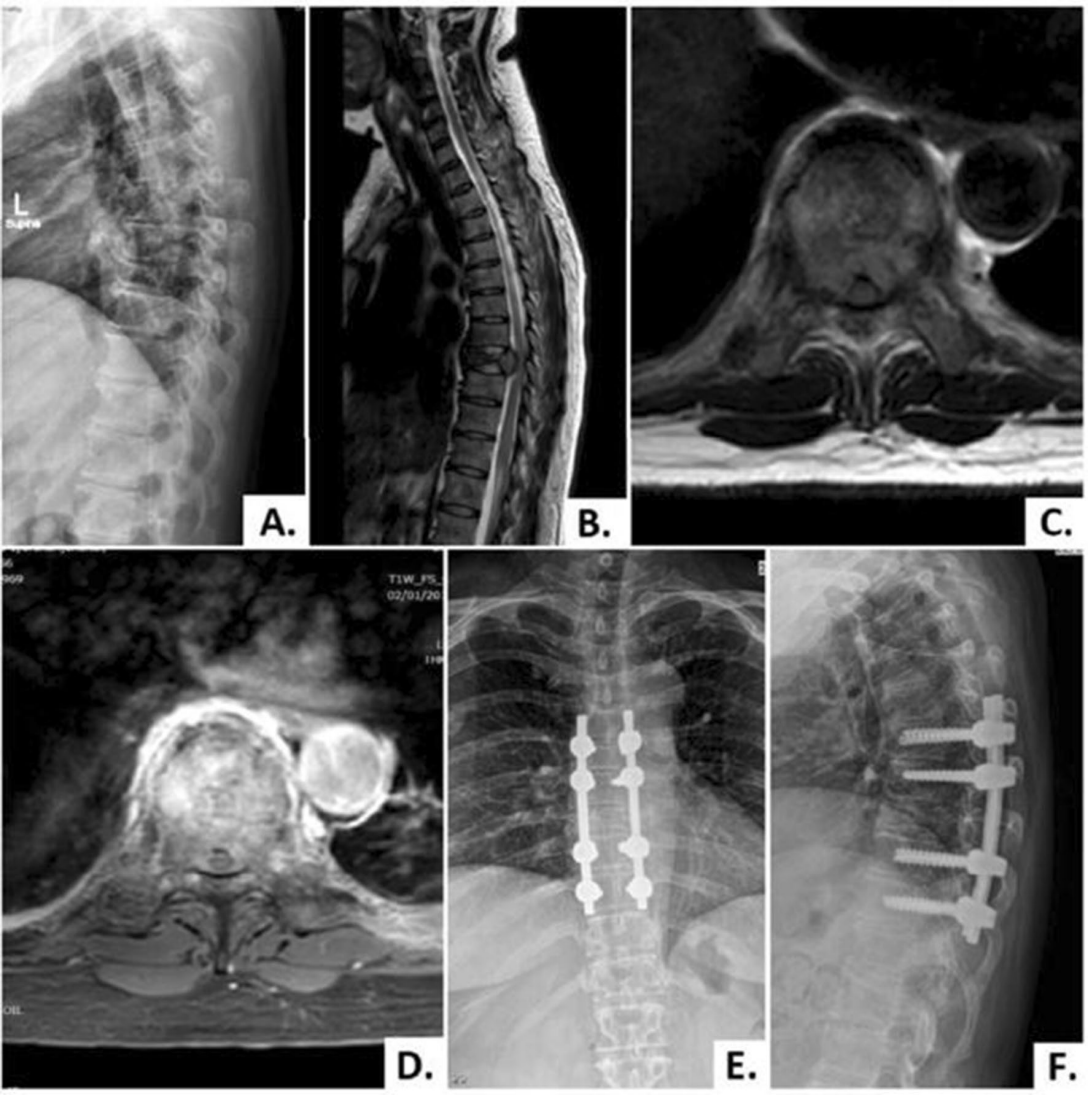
A 49-year-old female patient with breast cancer (case No. 15). (A, B) Lateral plain radiograph and sagittal T2-weighted MRI showed the collapse of the T8 vertebra and metastases tumor infiltration at the vertebral body with extension posteriorly into the spinal canal. (C, D) Axial T2-weighted and T1-weighted contrast-enhanced MRI showing enhancement of the vertebral body and both sides of pedicles with the metastatic tumor extension already encased and compressing the spinal cord. (E, F) Postoperative anteroposterior and lateral plain radiograph obtained at 3-month follow-up visit showing proper implant position without loss of fixation.
